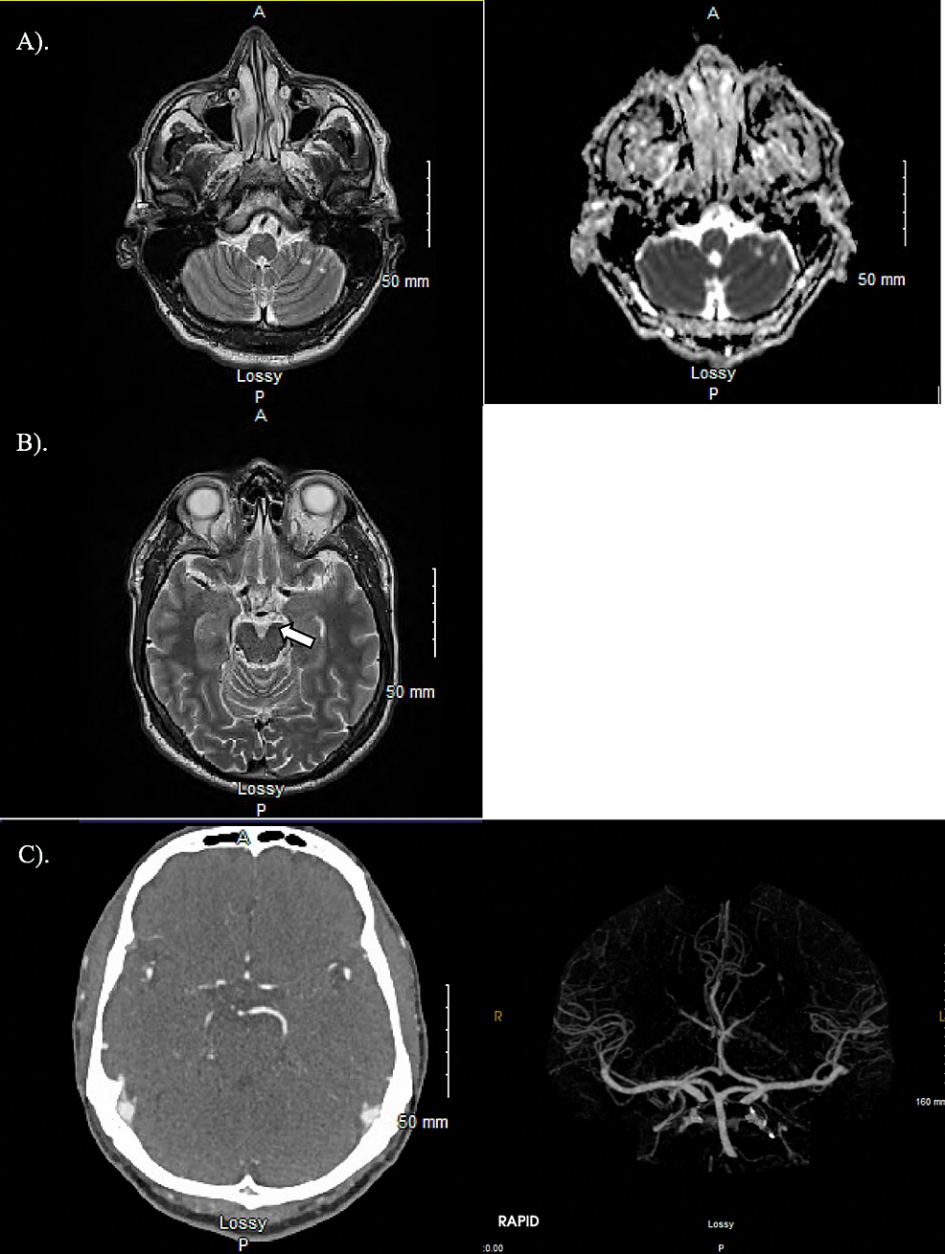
MRI imaging in a 45-year-old male with oculomotor nerve palsy and intractable nausea/vomiting A). Pre-contrast T2 axial MRI image with corresponding ADC diffusion sequence demonstrating two, small chronic left cerebellar infarct. B). Pre-contrast T2 axial MRI image demonstrating the absence of signal abnormality or compression left CN3 on imaging (see arrow) in a patient with left-sided ptosis. C). Computed tomography angiogram (CTA) head with and without contrast demonstrating normal-appearing posterior communicating arteries.
